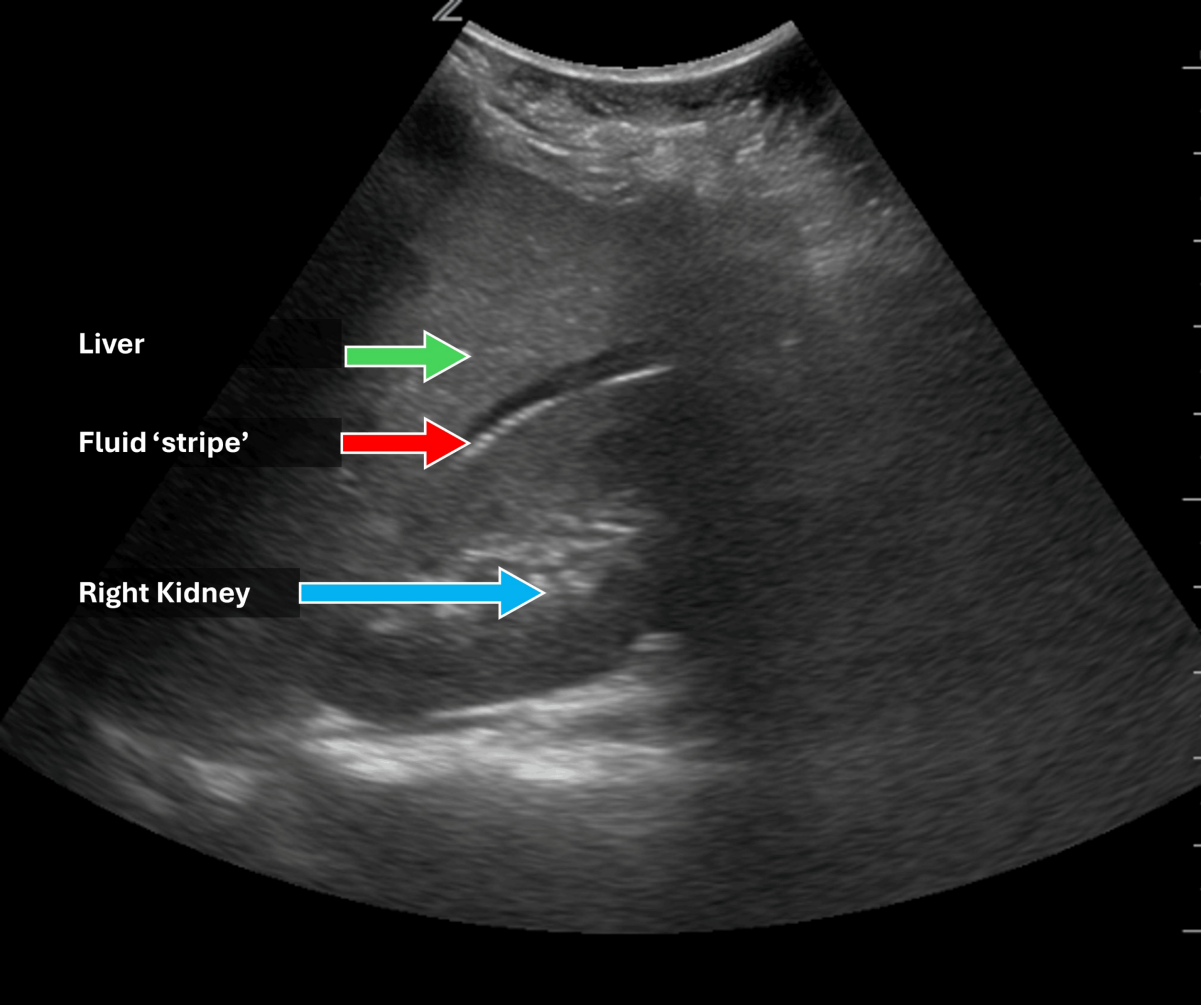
Focused abdominal sonogram for trauma (FAST) exam demonstrating intra-abdominal fluid, suggesting hemorrhage. The FAST exam is performed to examine multiple body cavities: cardiac, right upper quadrant, left upper quadrant, and pelvis to evaluate for free fluid. Here, an anechoic stripe between the right kidney and the inferior surface of the liver suggests intra-abdominal hemorrhage in the setting of hypotension after trauma.
